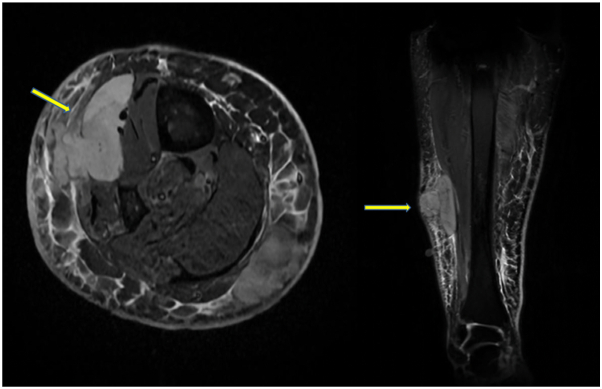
Magnetic resonance imaging scan of the lower portion of the right leg, demonstrating multiple soft tissue nodules within the subcutaneous tissue and muscles, spanning the facial planes, with the largest one measuring 9 cm. Heterogeneous intermediate signal on T2 image raised the suspicion of lymphoma.
